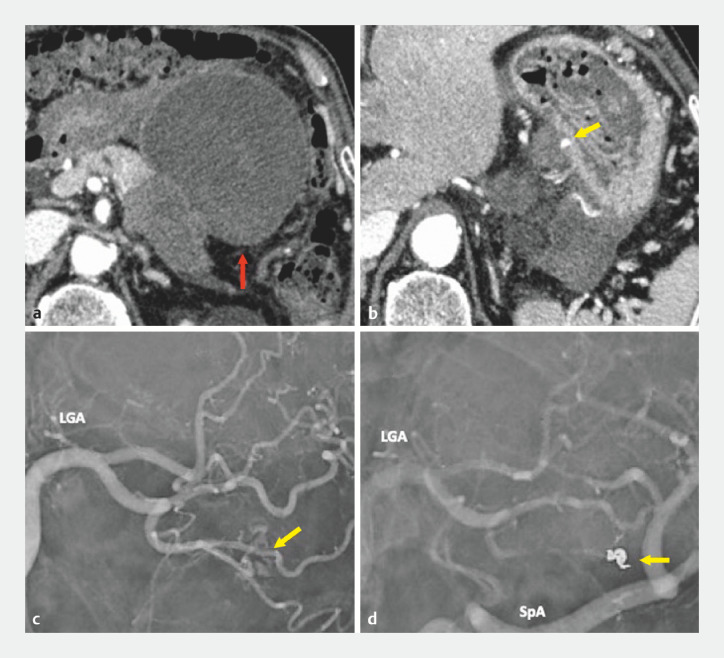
Contrast-enhanced computed tomography (CECT) image of hematoma and emergency transcatheter arterial embolization (TAE) image.
